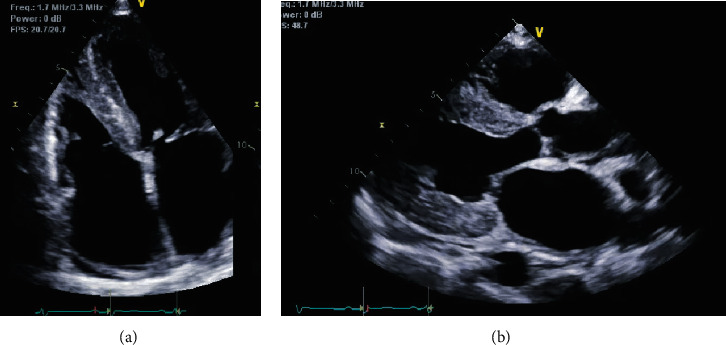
4-chamber (a) and a parasternal long axis echocardiogram view. Significant increased left ventricular wall thickness and biatrial enlargement can be seen.
